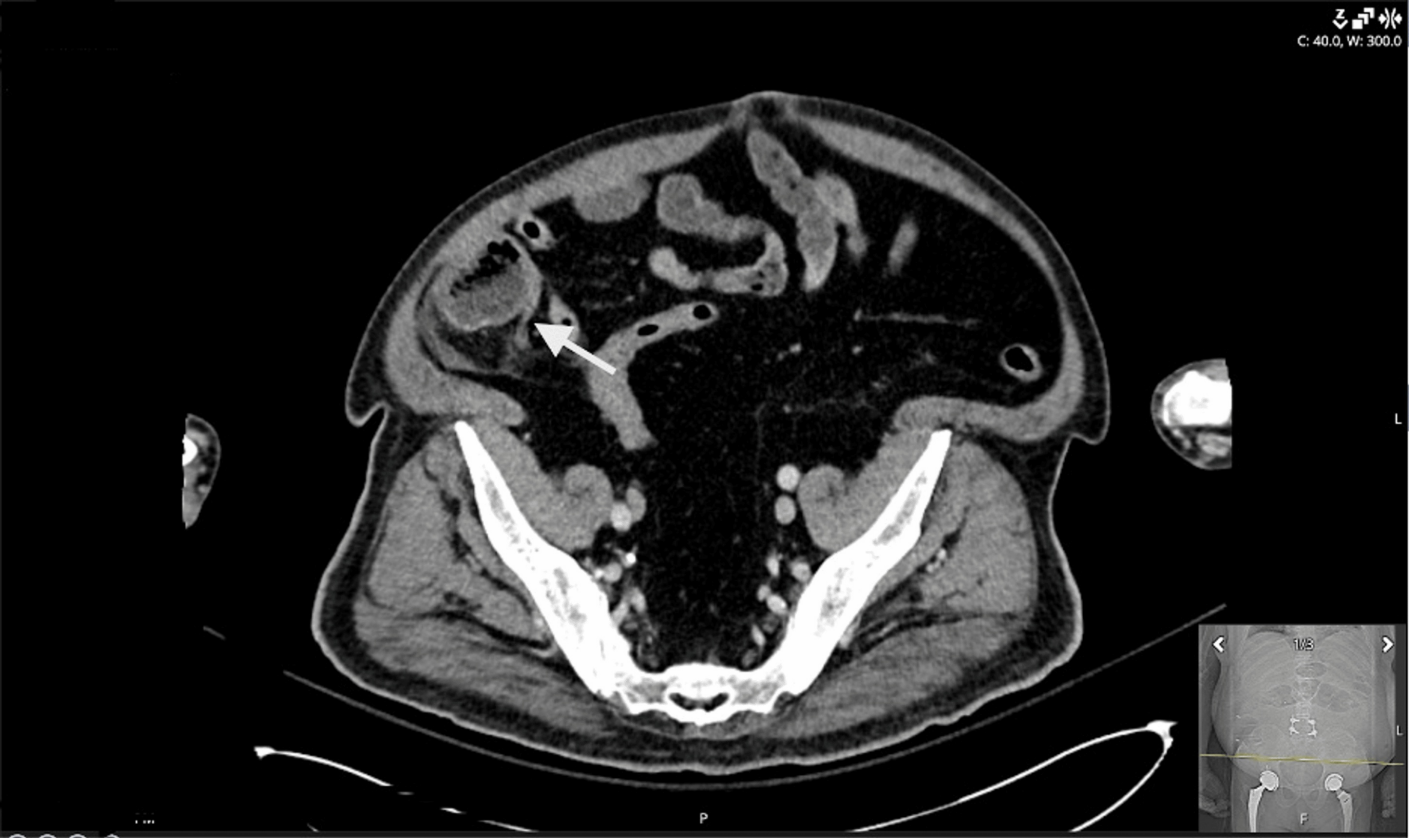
Contrast-enhanced axial CT of the pelvis demonstrating nonspecific right lower quadrant fat stranding adjacent to the appendiceal tip (white arrow).
